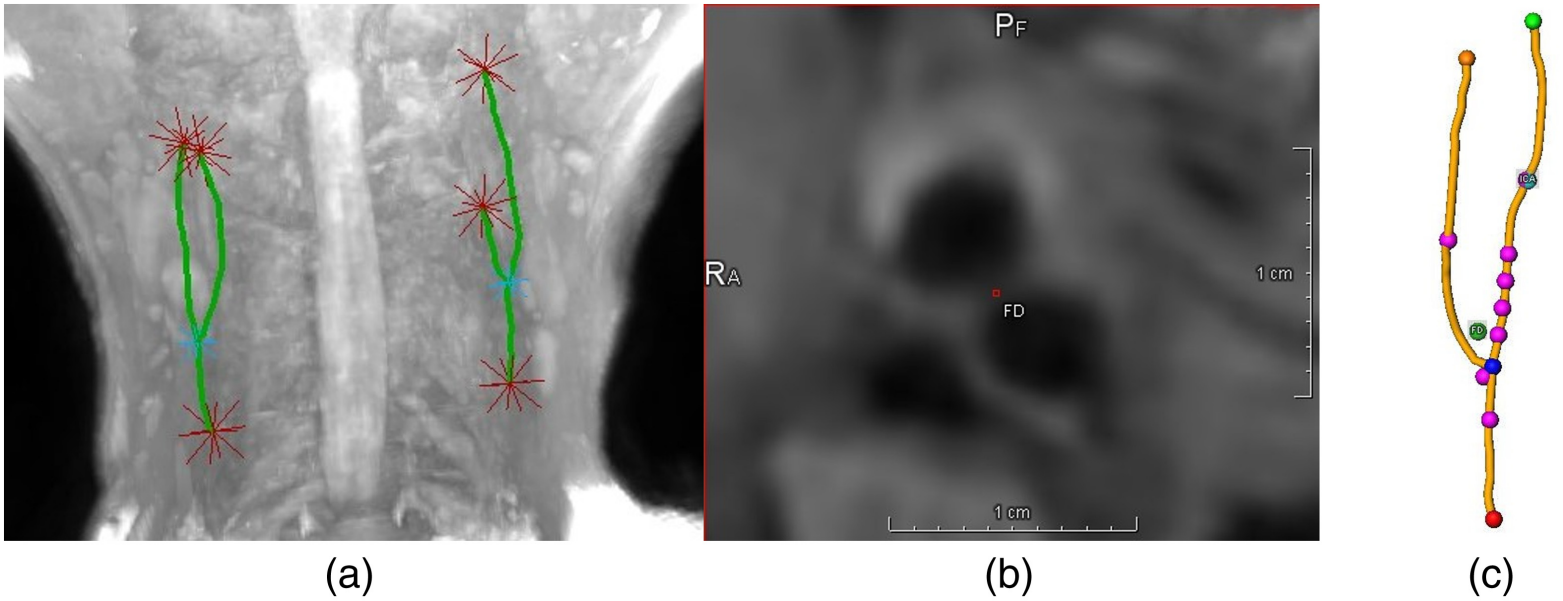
Plane placement with CaroTo: (a) A maximum intensity projection of the MRI volume with the centerlines of the carotid artery. The centerlines are generated automatically using four manually set points per artery. (b) Setting the FD for automatic plane generation. (c) Plane centers along the centerline graph. Positions are automatically generated based on manually set FD and ICA markers and the centerline graph.
